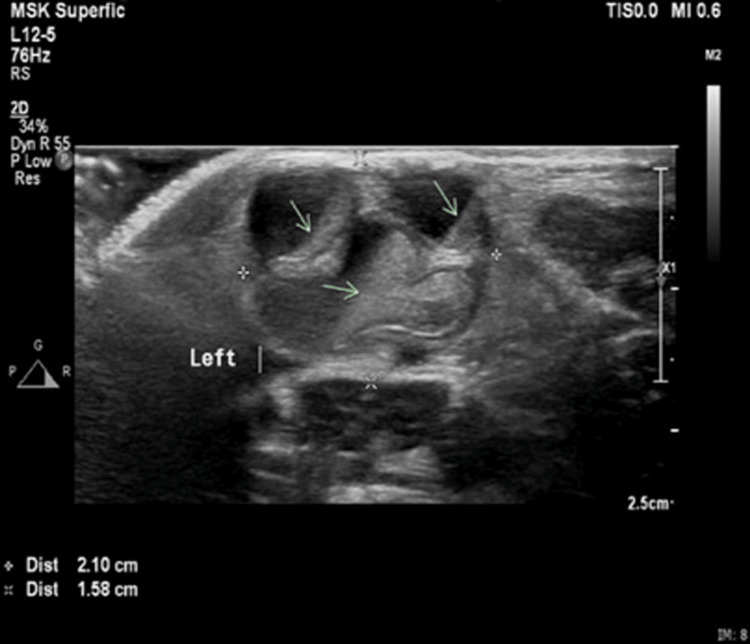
Ultrasound image of the swelling on the left side of the neck A grayscale ultrasound image taken at the level of the left posterior triangle of the neck showing a well-defined oval-shaped multiloculated cystic lesion (marked by calipers), revealing multiple fluid-debris levels (marked by arrows). Debris seen in the dependent part of each locule represents blood products/hemorrhagic contents. Note the lack of surrounding inflammatory changes.
